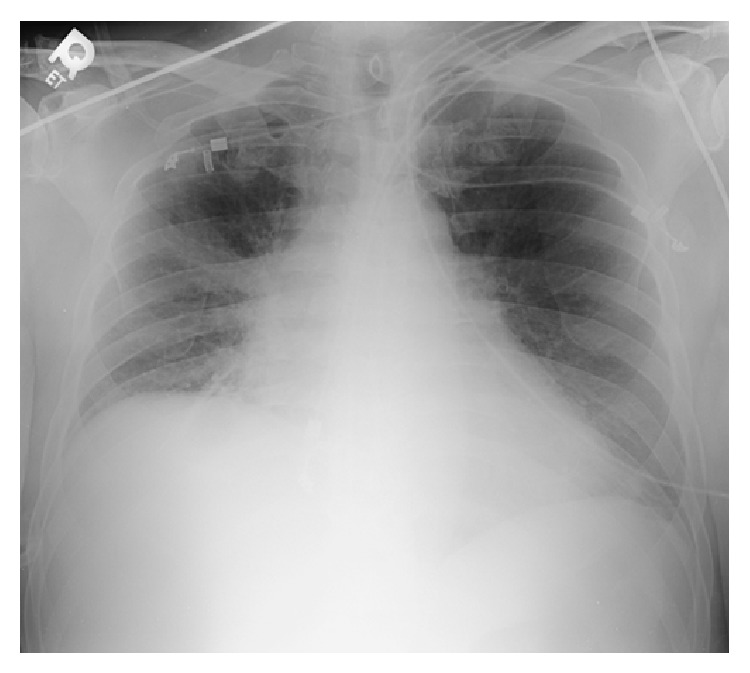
Chest X-ray AP view showing enlarged pericardial silhouette and right hilar mass.
